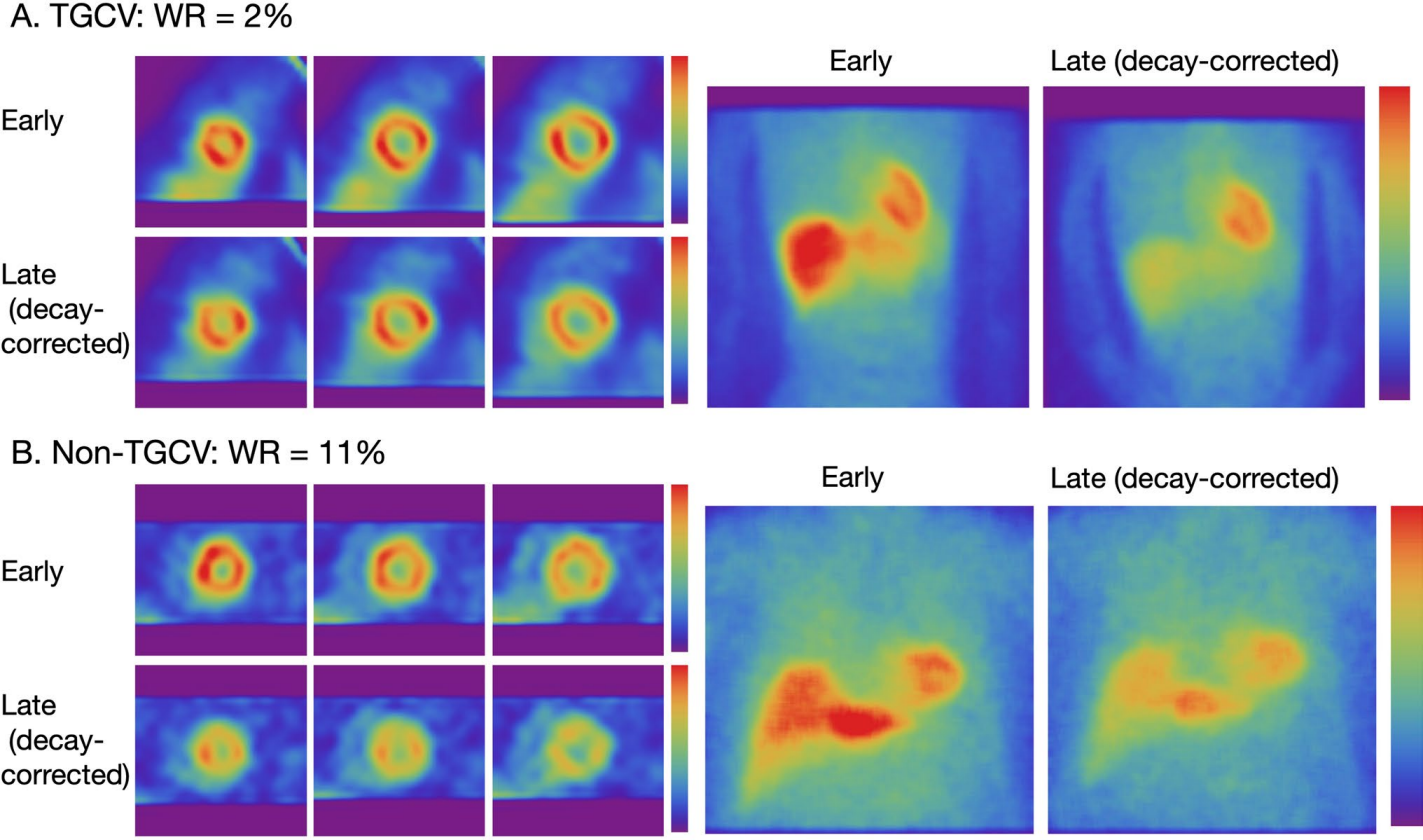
Three short-axis SPECT slices and anterior planar images of patients. A Patient with WR 2% and clinical diagnosis of TGCV. B Patient with WR 11%. Color scale of late images was corrected for time decay between early and late imaging. This can be achieved either by multiplying counts derived from late images by decay correction factor to the late image or modifying the maximum count in the late image adjusted for time decay. Correction for time decay led to similar heart counts between early and late images in patient A, whereas heart count was decreased in late image in patient B. *TGCV* triglyceride deposit cardiomyovasculopathy, *WR* washout rate
